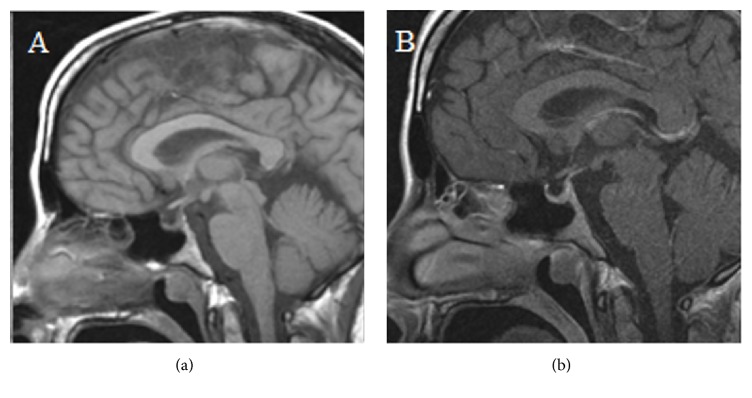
(a, b) Pre- and postcontrast brain MRI images of case 2 show that posterior pituitary bright spot is not visualized and that the anterior pituitary appears small for age and postpartum state.
